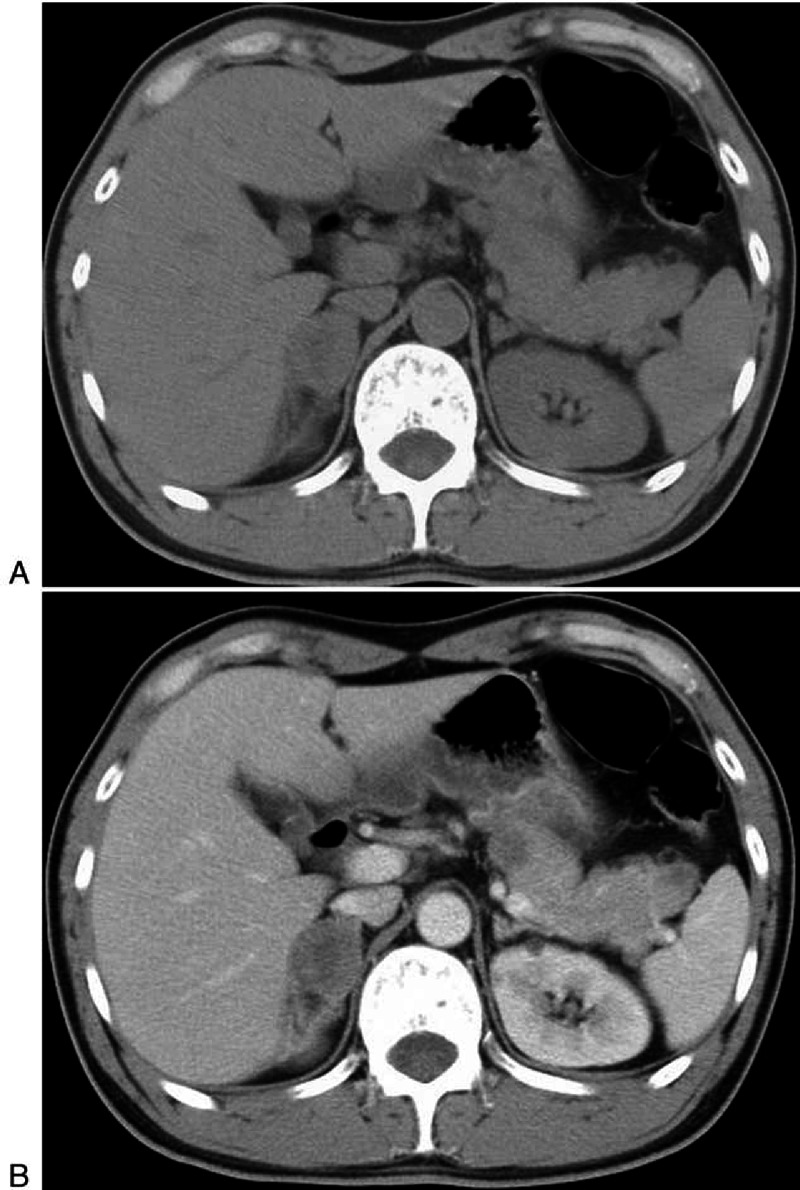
A 39-year-old man with NSCLC (patient 1). A, On unenhanced CT the metastases in pancreas are very inconspicuous. B, Contrast enhanced CT show multiple hypo-attenuating lesions with homogeneous enhancement. Right adrenal metastasis is also seen.
